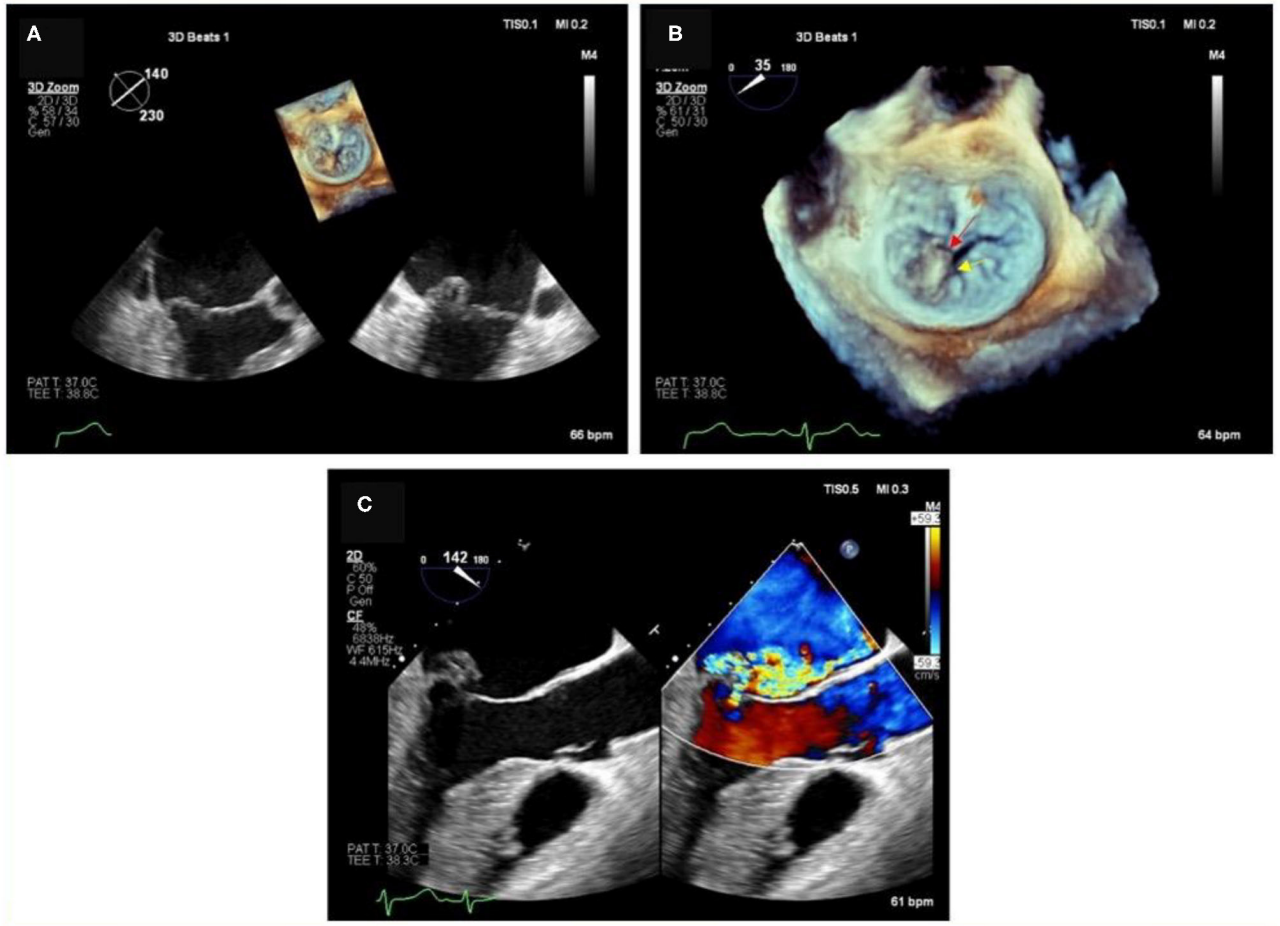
Degenerative MR due to P2 flail in the context of a complex anatomy. (A) 2D images and relative 3D (on top) showing P2 leaflet flail form the long-axis view and commissural view. (B) Magnification of the 3D short-axis on the MV (surgeons' view), where the P2 flail is easily appreciated (red arrow), together with a deep indentation (cleft- yellow arrow) between P2 and P3, which justify the presence of multiple jets. (C) Color Doppler image of the long-axis view, showing at least 2 regurgitant MR jets.
